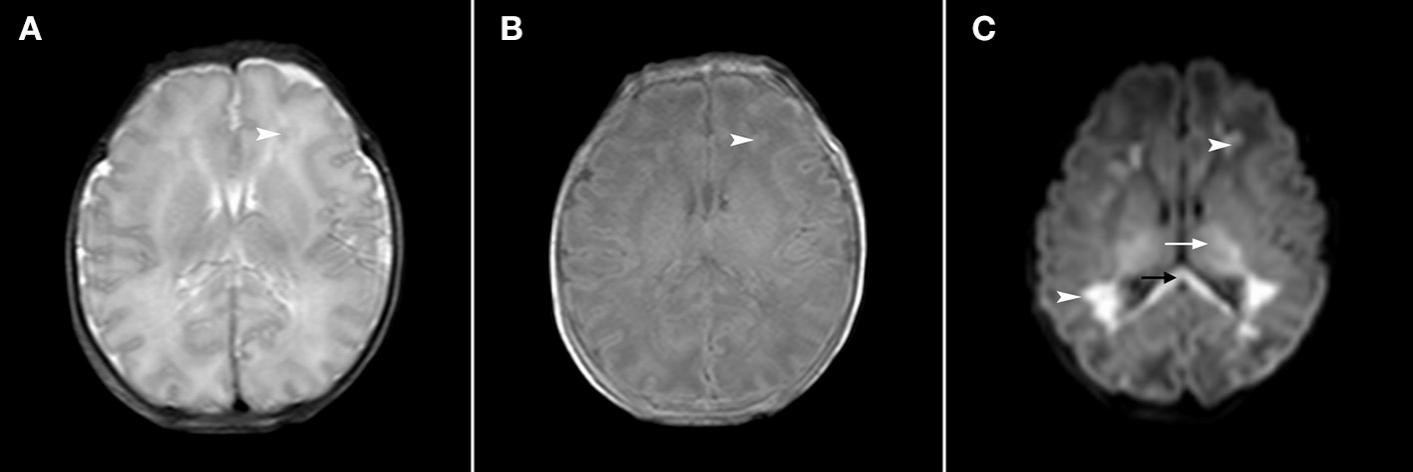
Axial T2-weighted, axial T1-weighted and DWI sections in a late premature neonate (gestational age, 35+5 weeks; 2 days after birth). In the T2-weighted image (A), the white matter signal of the prefrontal area was slightly decreased (arrowhead) and in the T1-weighted image (B), the signal was slightly increased (arrowhead). In the DWI (C), the signals of the prefrontal area and trigonometry were significantly increased (arrowhead), and the signals of the corpus callosum (black arrow) and thalamus (white arrow) were also significantly increased.
